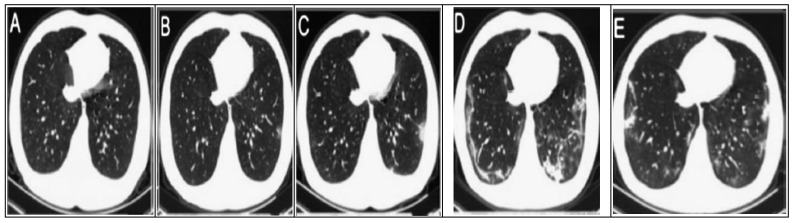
(A–E) subfigures show progression of a CT scan of a COVID-19 patient across days (2, 4, 5, 6, and 8, respectively).
